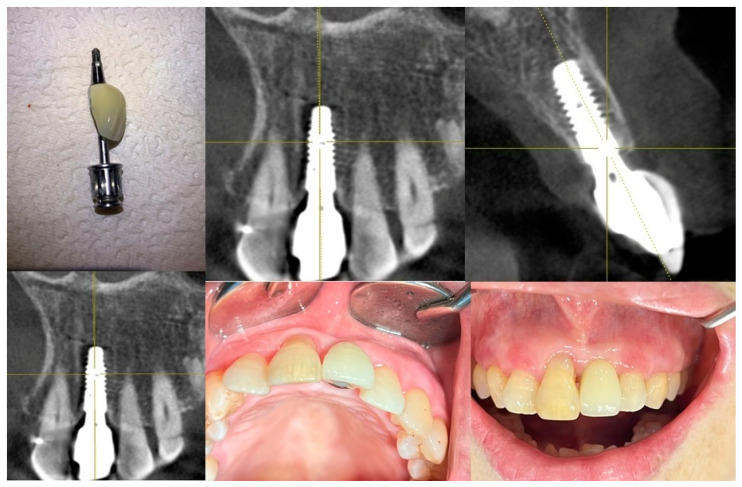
Although the plain X-ray image suggests that the implant was placed subcrestally, the CBCT image reveals that the fixture was actually placed epicrestally in the buccolingual aspect. This discrepancy highlights the limitations of two-dimensional imaging, emphasizing the need for three-dimensional evaluation to accurately assess implant positioning relative to the crestal bone in both the buccolingual and mesiodistal dimensions.
